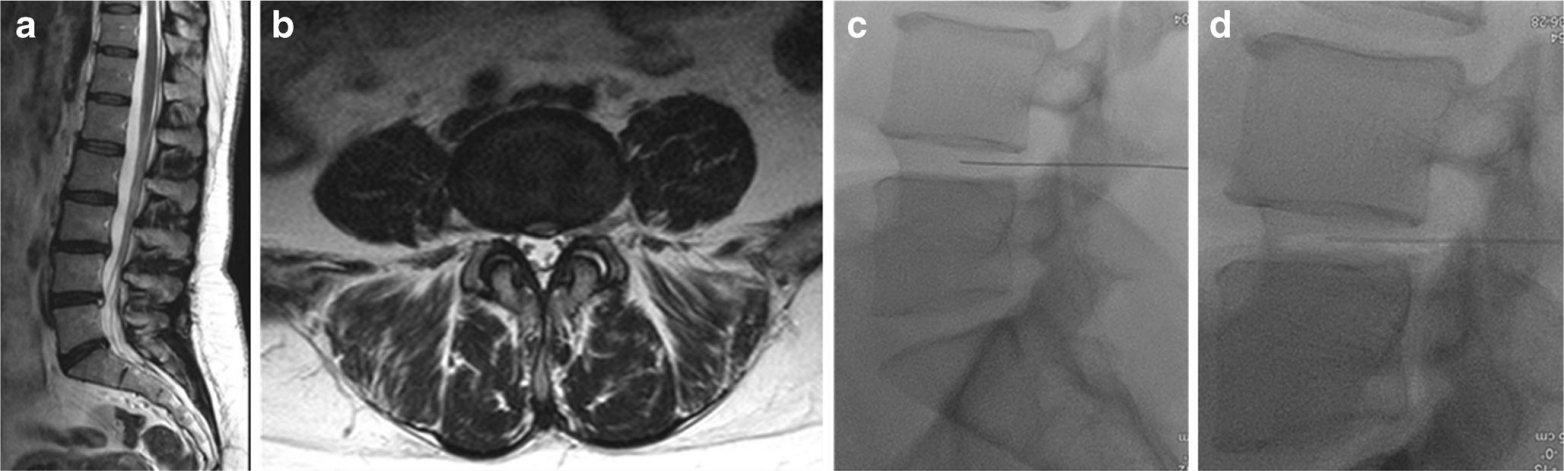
A 43-year-old women presented with lumbalgia and treated by of O_2_-O_3_ discolysis. a MRI T2-weighted sagittal image shows diffuse degenerative changes with small disc protrusion at the level of L4–5, associated with a high signal annular tear. b The corresponding axial MRI T2-weighted image shows a left para-median disc protrusion, minimally indenting the dural sac, at the aforementioned level. c, d Lateral fluoroscopic images of the same patient show the needle placed inside the disc center as well as the distribution of O_2_-O_3_ mixture (d) after the injection that displayed as a faint white opacity inside the disc and epidural space
